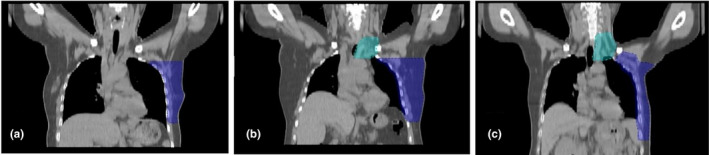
The coronal view of the computed tomography (CT) images and structure for the patients (a) the first situation (chest wall only), (b) the second situation (chest wall included supraclavicular lymph nodes), and (c) the third situation (chest wall included supraclavicular and axillary lymph nodes irradiation).
